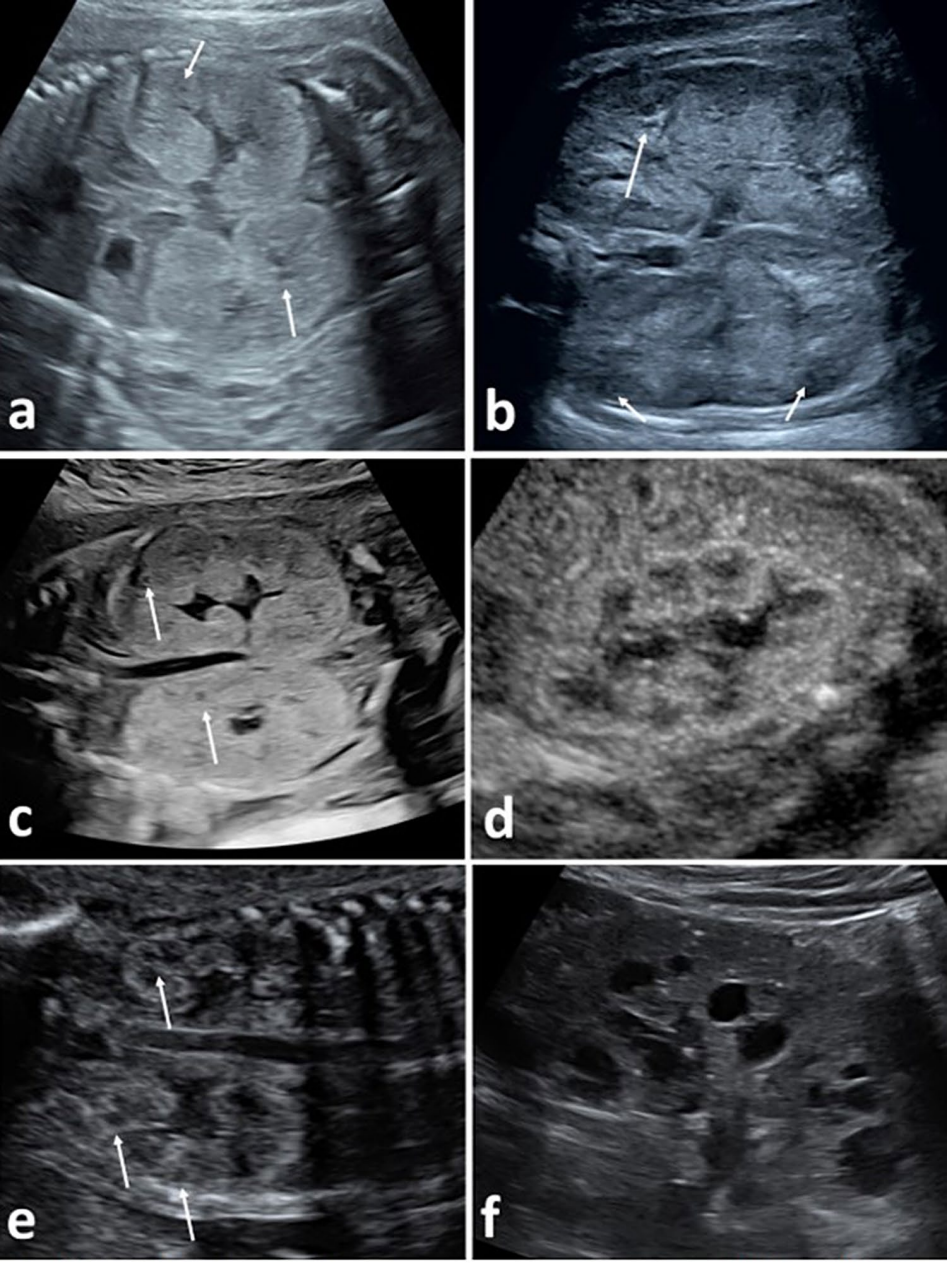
Prenatal ultrasound in autosomal recessive polycystic kidney disease (*ARPKD *a, b) and autosomal dominant polycystic kidney disease (*ADPKD*, c–f). a shows fetal kidneys in *ARPKD* at 23+3 weeks’: kidneys present enlarged, hyperechogenic and with absent corticomedullary differentiation (CMD); small cysts can be seen in the medulla (white arrow); cysts show a variable size from 1 to 9 mm and a relative increase in size with advancing gestational age; b shows the kidneys of another fetus with *ARPKD* at 30+5 weeks’: kidneys are enlarged with reverse *CMD* (hyperechogenic medulla and hypoechogenic cortex). In this case, cysts (white arrow) are predominantly located in the cortex, however in most cases of *ARPKD* they can be seen in both cortex and medulla; c shows kidneys of a fetus with *ADPKD* at 24+0 weeks’: in this case, kidneys are enlarged, hyperechogenic with absent corticomedullary differentiation (*CMD*) and small cysts (white arrows); d kidney of another fetus with *ADPKD* at 28+0 weeks’, showing enhanced CMD; e shows the kidneys of another fetus with *ADPKD* at 24+1 weeks’: *CMD* is preserved with visible cysts (white arrows) predominantly located in the cortex; f shows the kidney of the mother of the fetus in e with multiple cysts in both cortex and medulla.
